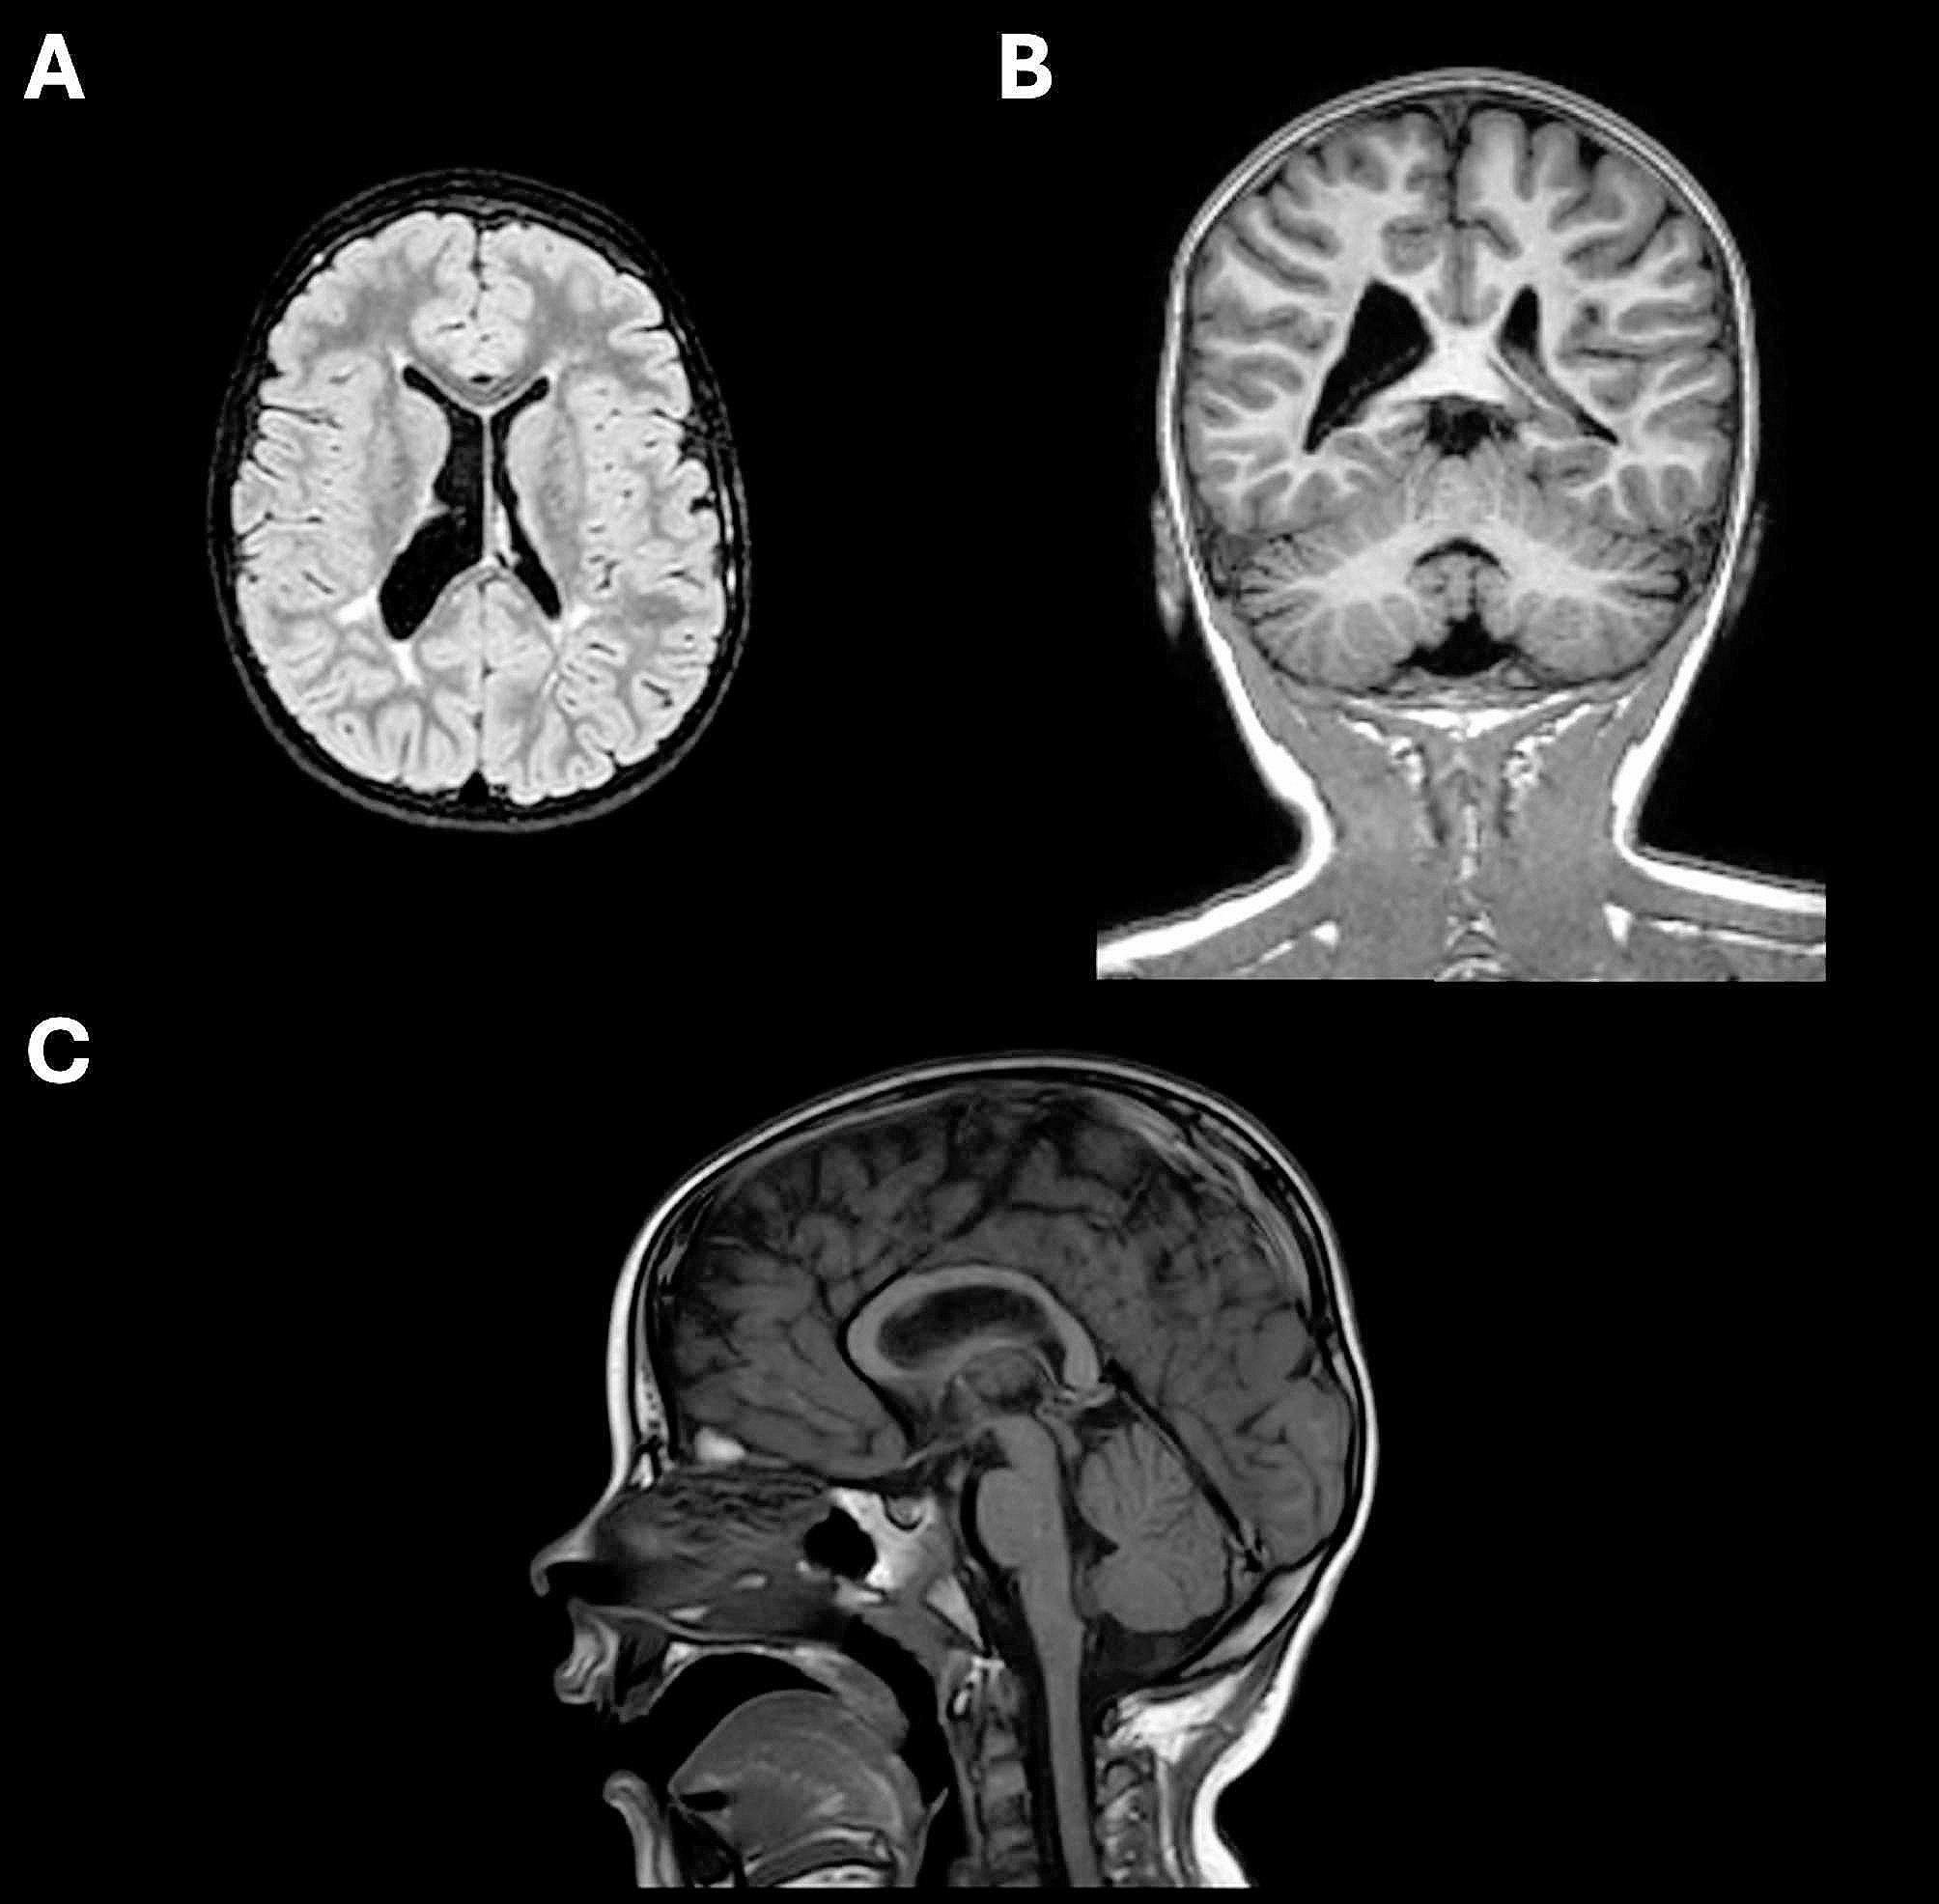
Encephalic MRI scans comprehensive of a T2-FLAIR axial view (A), a T1-weighted coronal view (B), and a T1-weighted sagittal view (C). Neuroimaging reveals asymmetrical enlargement of the right ventricle, slight enlargement of the periencephalic liquor spaces in the left temporal pole, periventricular white matter thinning and gliosis, and corpus callosum abnormalities in posterior brain regions
